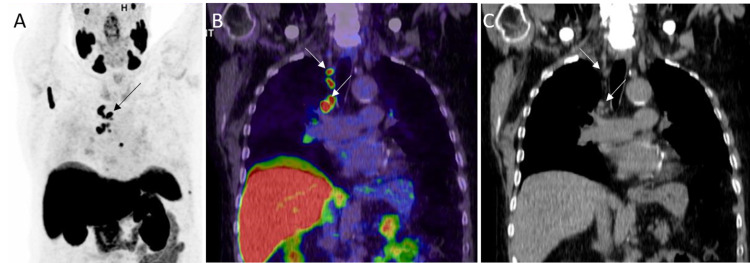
Metastases in mediastinal lymph nodes A: 68Ga PSMA PET MIP image shows intense uptake in the metastatic mediastinal lymph nodes (black arrow). B: Coronal PSMA PET/CT image through the chest and upper abdomen of the same patient shows intense radiotracer uptake in the mediastinal lymph nodes (white arrows). C: Coronal CT image shows the correlate mediastinal lymph nodes (white arrows) that are metastatic.
